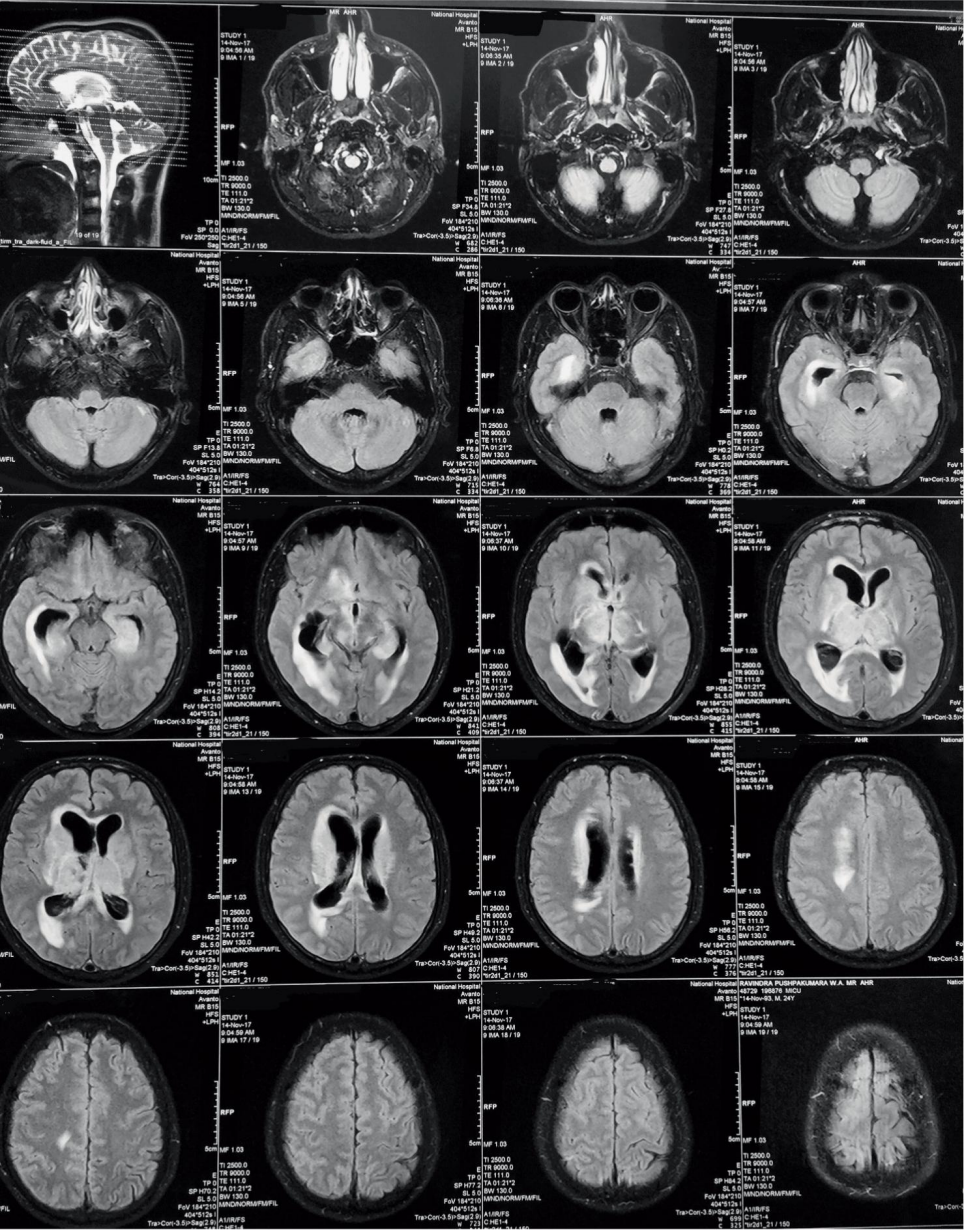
MRI showing hydrocephalus, periventricular oedema and leukoaraiosis.
